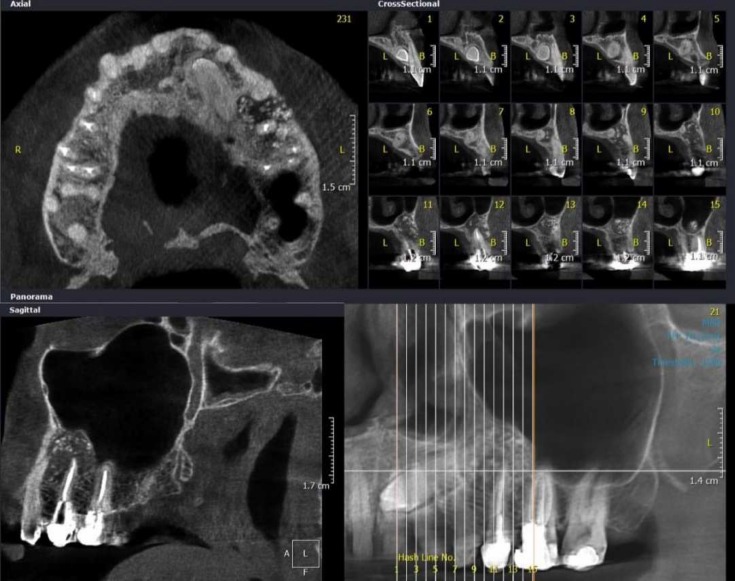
Preoperative cone-beam computed tomography evaluation: axial and cross sectional sections (above) and sagittal views (below) of the extending up periradicular lesion surrounding the root of tooth #25; the presence of bone replacement materials is noticeable
